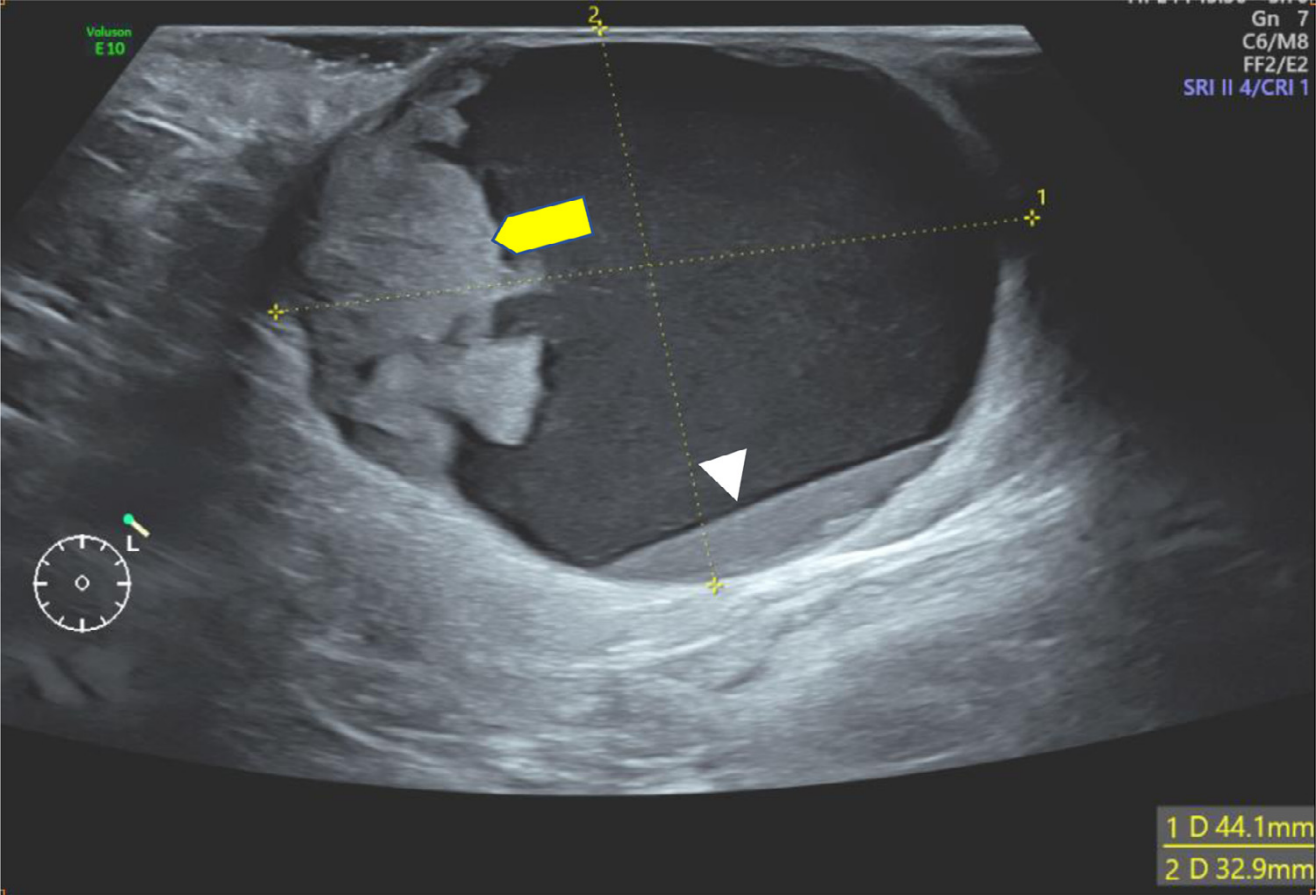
Ultrasound of the left axilla showing a complex cystic and solid mass, containing an echogenic-anechoic liquid level (head arrow), and a tissue component (yellow arrow).
